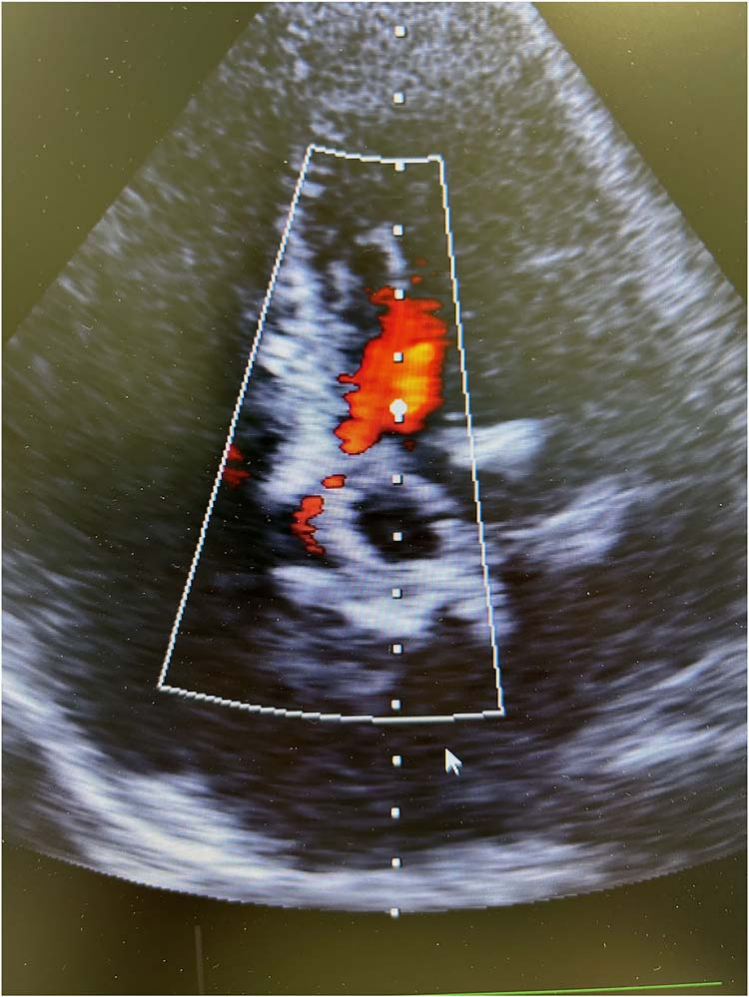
Postoperative transesophageal echocardiography showing nonsignificant regurgitation of a well-seated rapid-deployment Edwards Intuity bioprosthesis.
